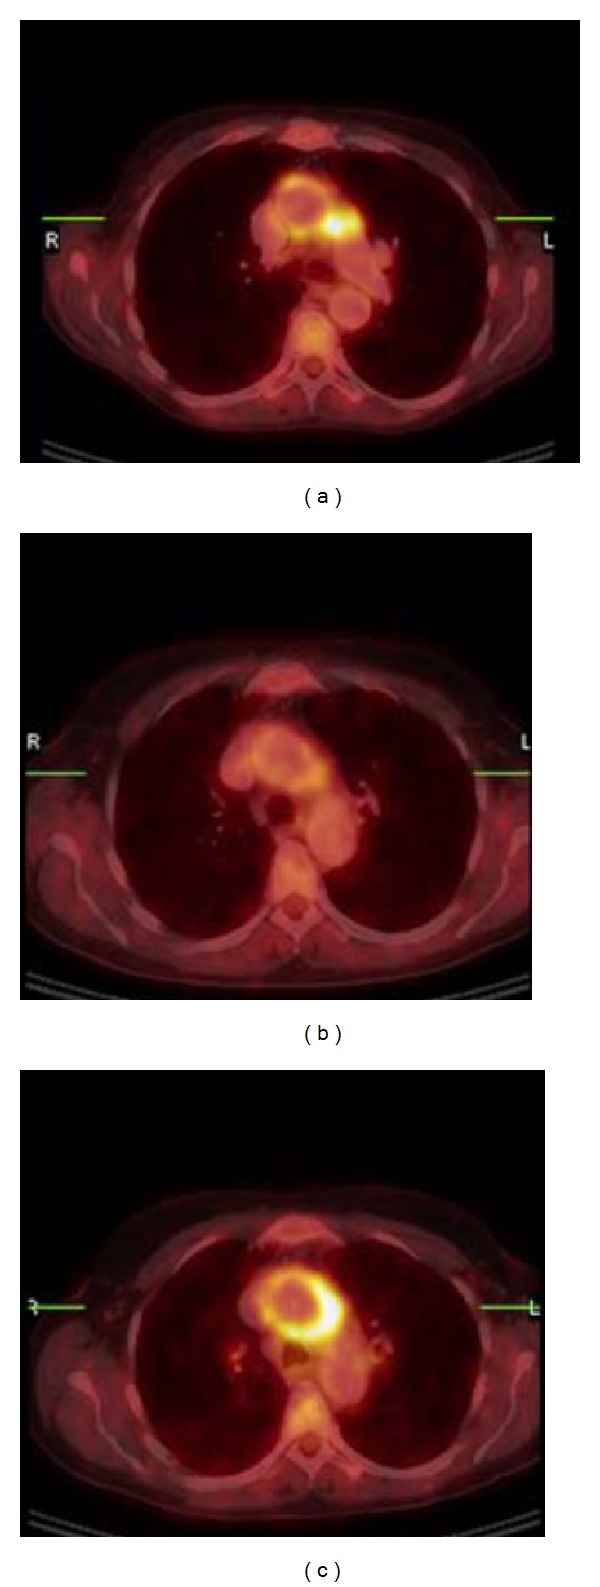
Aortitis in Cogan's syndrome. (a) Transverse hybrid PET/CT slice; pathological uptake in the wall of the aortic arch, more intense in the lateral wall and perivascular space adjacent to the truncus pulmonalis. (SUVmas 12, ESR 52 mm/h, CRP53 mg/L). (b) Follow-up PET/CT showed clearly decreased uptake in the aortic arch after 3 weeks treatment with methyl-Prednisolon i.v. and Prednisolon orally. (SUVmax 4, ESR 11 mm/h, and CRP < 2 mg/L). (c) Second follow-up PET/CT 6 months later (patient was in a stable condition with methotrexate and low-dose prednisone) with again high uptake in the wall of the aortic arch, with higher intensity in the lateral wall and perivascular space adjacent to the truncus pulmonalis. Methotrexate and prednisone were both increased to 20 mg/day (SUVmax 13, ESR 24 mm/h, and CRP 14 mg/L).
